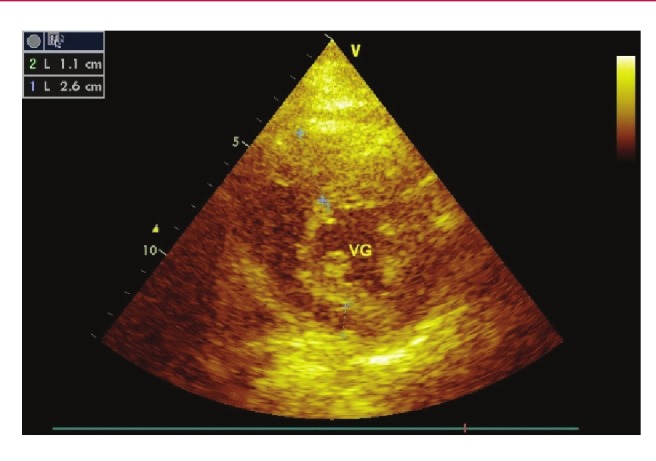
Image d’échographie cardiaque coupe parasternale petit axe d’un patient de 27 ans porteur de cardiomyopathie hypertrophique. Notez l’hypertrophie septale (26 mm) par rapport à la paroi postérieure (11 mm).
